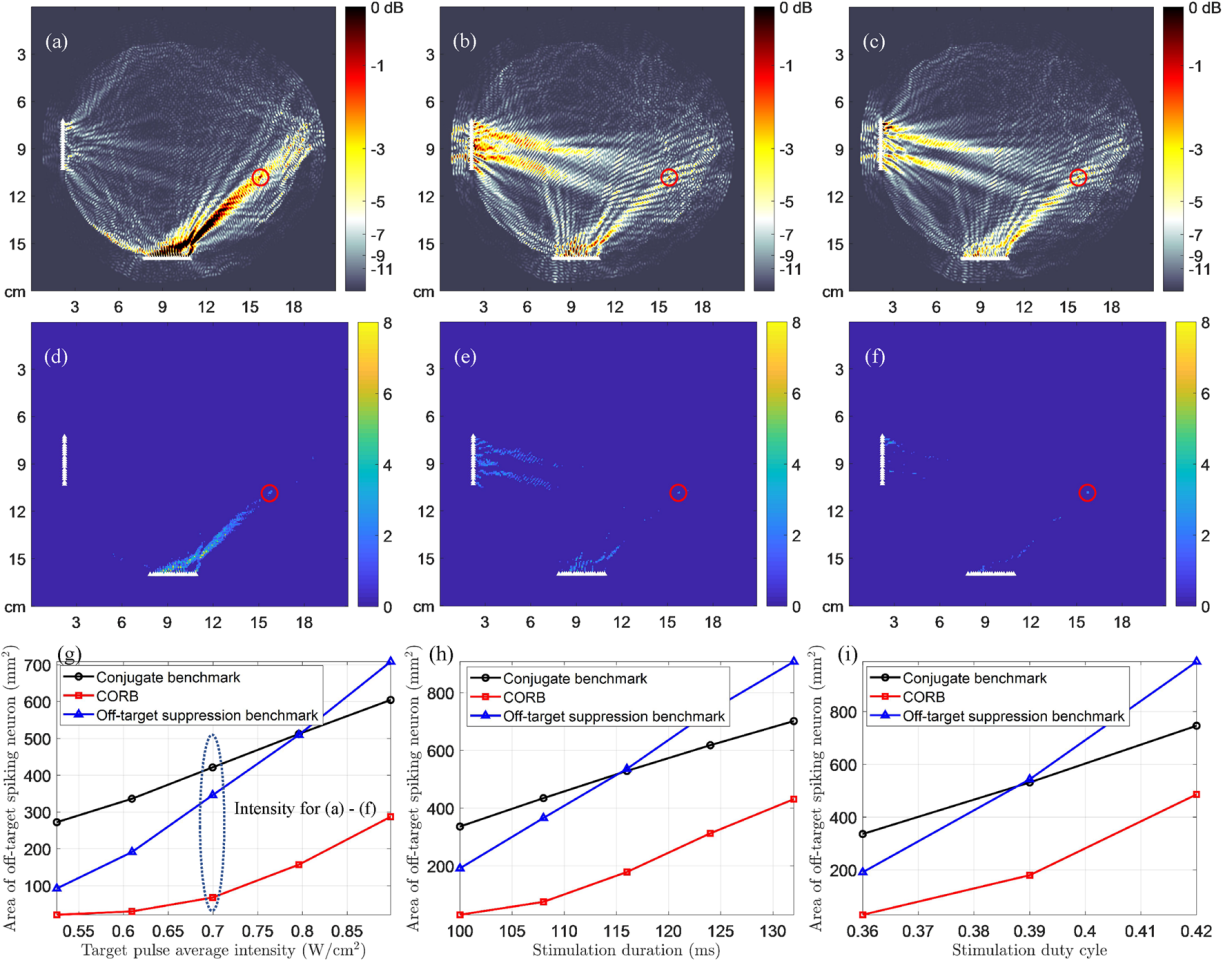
The beam patterns and neuronal spike patterns of intracranial stimulation using double transducer arrays, demonstrating the minimized OTAA of CORB compared with two benchmarks. The distance from the target point to the bottom array is approximately 8.18 cm, and the distance to the left array is approximately 13.73 cm. (a–c) Approximate beam patterns during a pulse of $$ T = 0.36 $$ ms generated by the conjugate benchmark, the off-target suppression benchmark, and CORB, respectively^3^. The color maps demonstrate the spatial distribution of mean ultrasound intensity normalized by the target signal intensity in the pulse (0.70 W/cm$$^{2}$$)^22^. (d–(f)) Neuronal spike count map of conjugate benchmark, the off-target suppression benchmark, and CORB, respectively, in a pulsed-wave LIFU stimulation (target intensity: 0.70 W/cm$$^{2}$$; duration: 100 ms; duty cycle: $$ 36 \% $$; PRF: 1 kHz). Ultrasound pressures at all other points are scaled proportionally to the target based on the mean intensity distributions in (a–c). (g) The OTAA with different stimulation intensities (duration: 100 ms; duty cycle: $$ 36 \% $$; PRF: 1 kHz). (h) The OTAA with different stimulation durations (target intensity: 0.61 W/cm$$^{2}$$; duty cycle: $$ 36 \% $$; PRF: 1 kHz). (i) The OTAA with different duty cycles given the fixed pulse duration (target intensity: 0.61 W/cm$$^{2}$$; duration: 100 ms).
